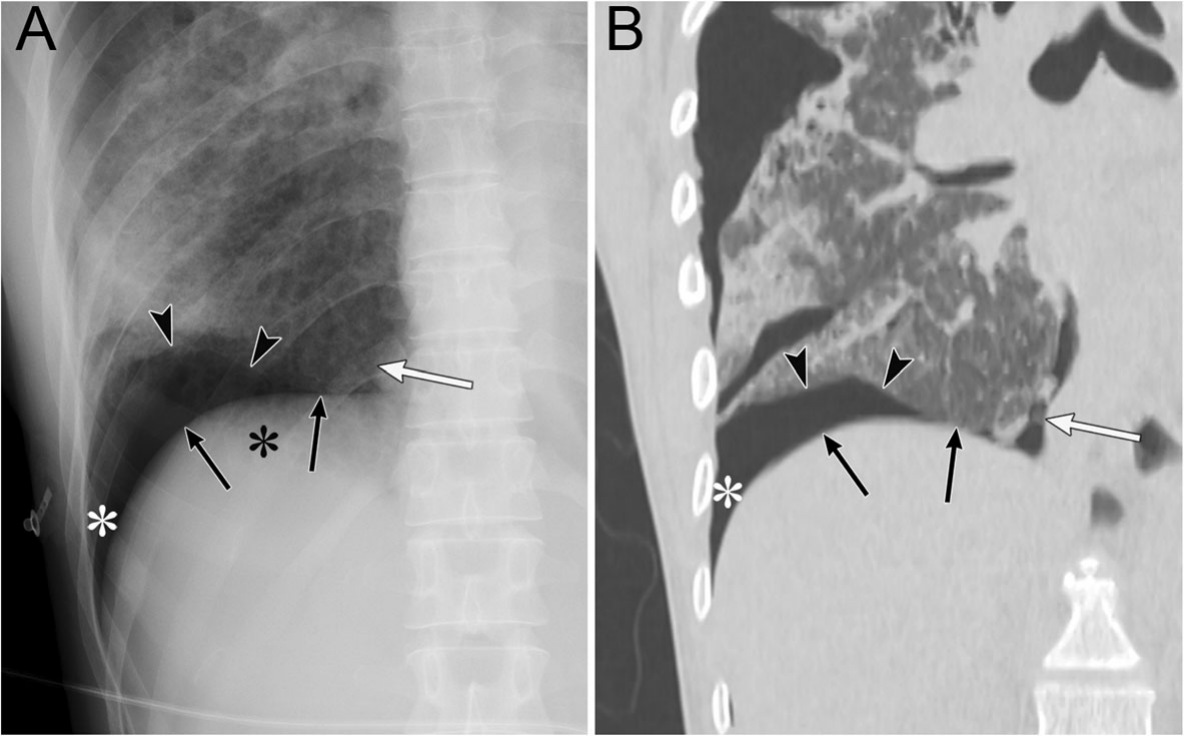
Pneumothorax in the subpulmonary space of a 36-year-old woman with interstitial pneumonia. a A supine radiograph shows a hyperlucent upper quadrant of the abdomen (black asterisk). The inferior surface of the lung (arrowheads), a sharply outlined diaphragm (black arrows), the sharp delineation of the inferior vena cava (IVC) (white arrow), and the “deep sulcus” sign (white asterisk) are also visible. b A coronal CT image clearly depicts the inferior surface of the lung (arrowheads), a sharply outlined diaphragm (black arrows), the sharp delineation of the IVC, and the “deep sulcus” sign (white asterisk)
